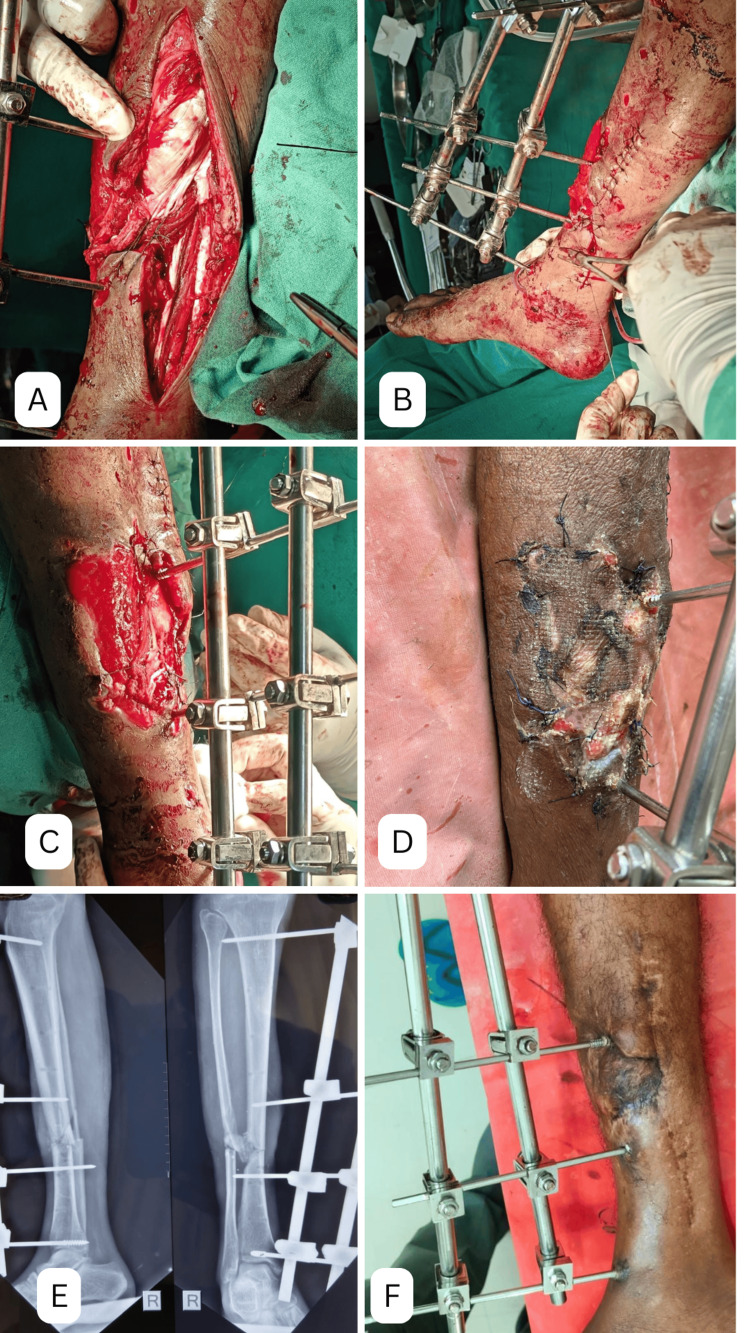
(A) Combined medial gastrocnemius and hemisoleus flap cover. (B) Closure of medial incision for taking flap. (C) Bone covered with combined medial gastrocnemius and hemisoleus flap and granulation tissue over anterior compartment muscle prior to putting a split skin graft. (D) Split skin graft applied over flap and granulation tissue (post-operative day 5). (E) Immediate post operative anteroposterior and lateral radiographs. (F) Flap cover at six weeks. These images correspond to Case 1 from the study.
